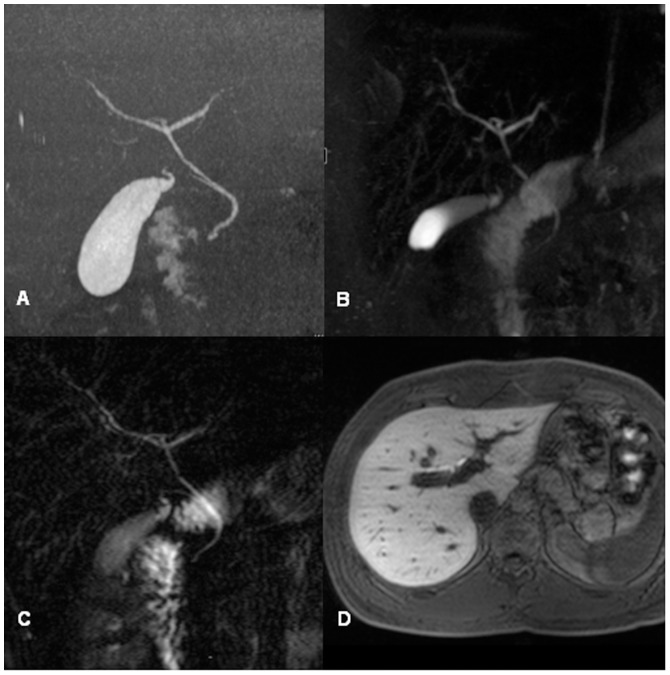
Comparison of MRC sequences in a 23-year-old male potential living liver donor with a central trifurcation (Smadja & Blumgart Type B) seen in contrast enhanced CT cholangiography (A). 3D TSE RESTORE MIP (B), thick slab 2D HASTE (C) and Gd-EOB-DTPA enhanced 3D T1w MRC without IR (D) are all able to detect this variant anatomy.
